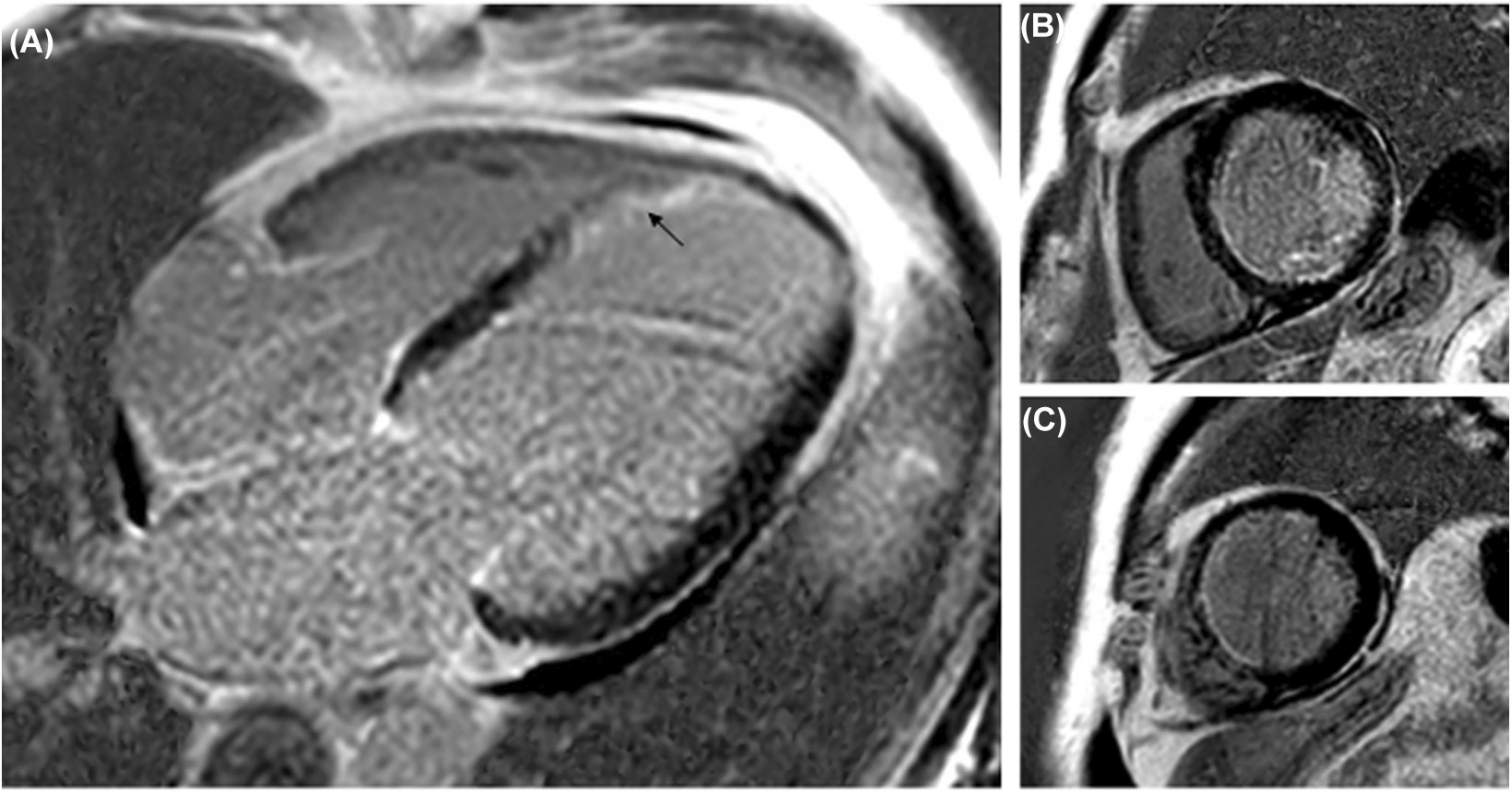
Viability assessment with cardiac MRI: Panel (A) shows late gadolinium enhancement (LGE) acquisition in four Chamber plane with a transmural infarction at distal apical septal segment (see arrow). Panel (B) shows LGE images in short axis plane with mid inferoseptal and mid inferior subendocardial infarction. Panel (C) shows proximal apical septal segments with subendocardial infarction. These findings were consistent with viable RCA and circumflex territories and predominantly viable LAD, with non‐viable distal apical septal segment.
